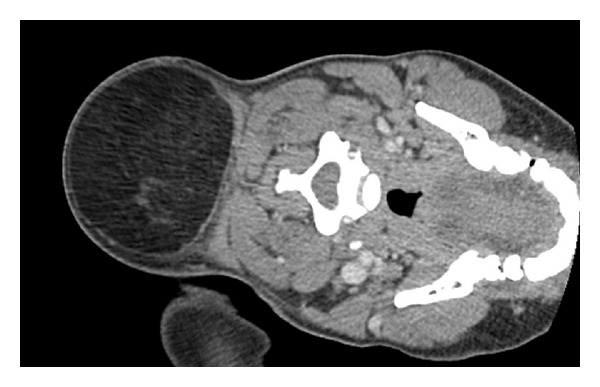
CT image showing a slice of a large posterior cervical lipoma which spanned from the patient's occiput through the length of the patient's cervical spine.
